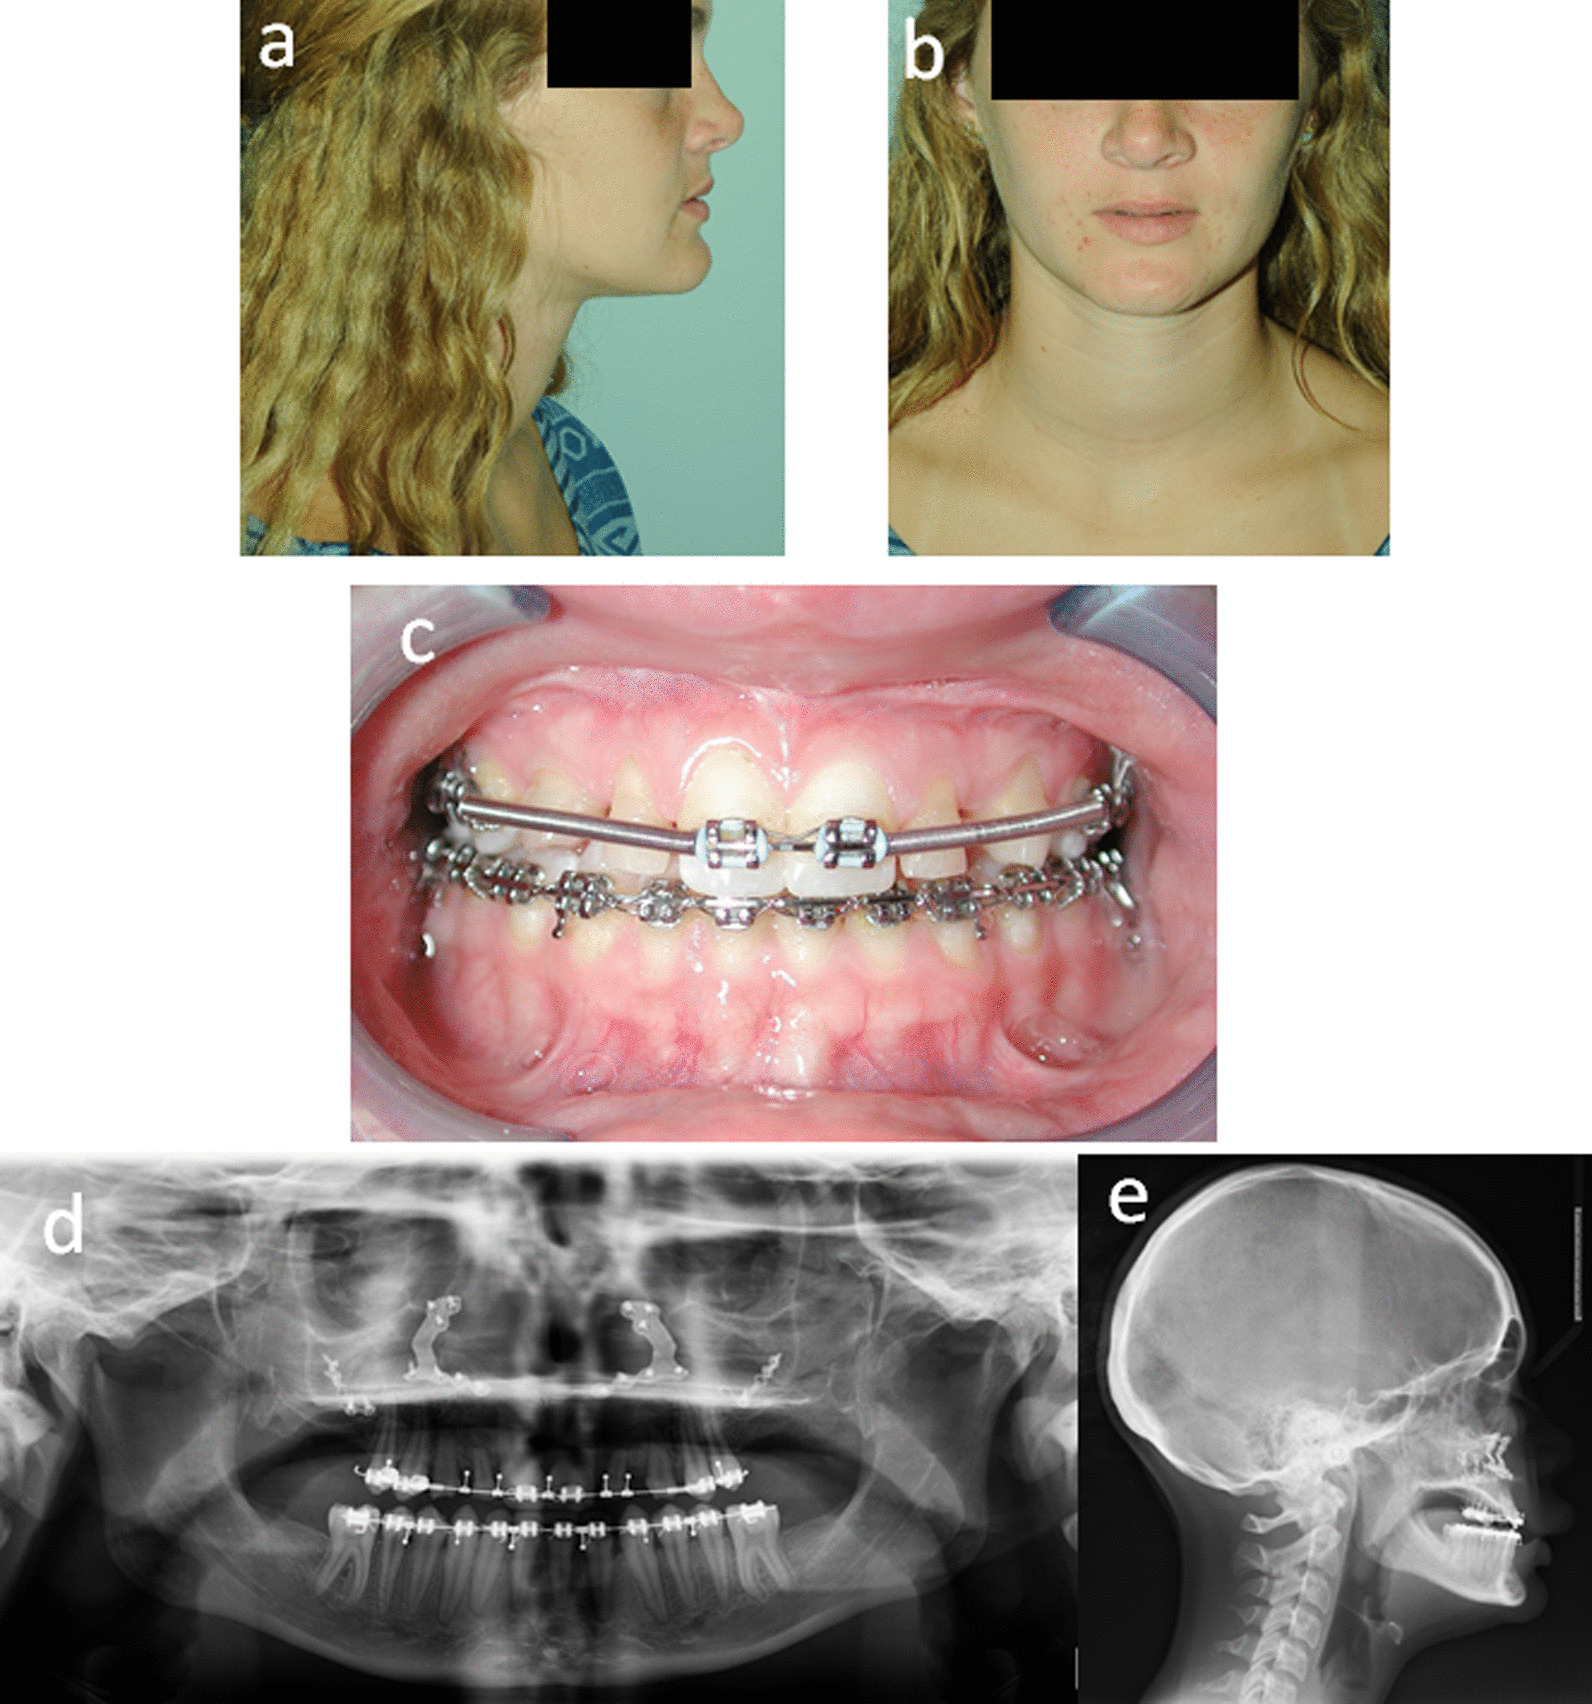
After the high Le Fort 1 maxillary orthognathic surgery: a and b Extraoral photograph. c Intraoral photograph. d Panoramic radiograph. e Cephalomatric radiographs
